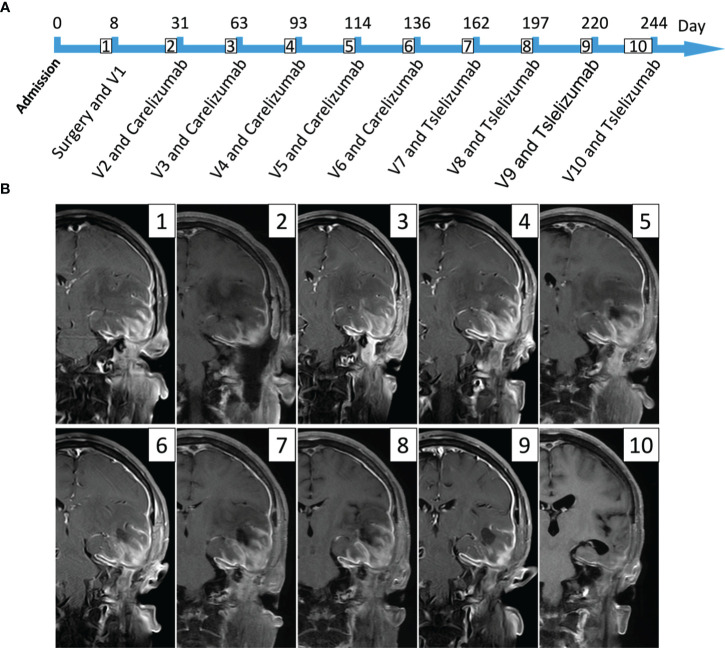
This figure mainly expresses the timeline of the patient’s treatment and the lesion changes in imaging. (A) The patient received a total of one intraoperative oncolytic virus treatment and then nine oncolytic virus and PD-1 inhibitor treatments. The first five times used 110 mg of carelizumab, and the last four times used 200 mg of tislelizumab; the dose of oncolytic virus used was 5 × 10^11^vp (V represents the number of virus treatments). (B) It mainly shows the changes in the patient’s MRI scans during treatment (T1 enhancement phase); the preoperative images (1) can see the tumor lesion, partly invading the surrounding soft tissues and dura mater; the left temporal lobe and insular edema are very severe. The postoperative MRI shows that the tumor in the left temporal region has been sub-totally removed, but the part that erodes the dura mater and surrounding soft tissues still exists. After using the combined treatment nine times, the last follow-up image (10) can hardly see the enhanced image, and the patient’s symptoms are almost completely relieved.
